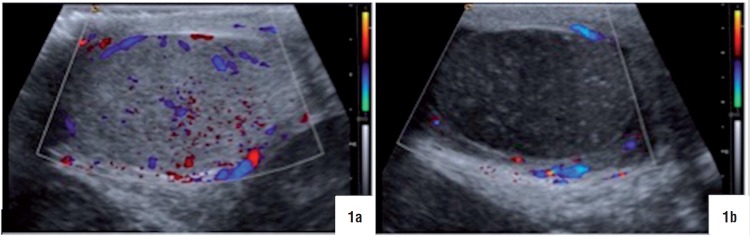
Doppler ultrasound in the initial evaluation. a) right testis. b) left testis.
